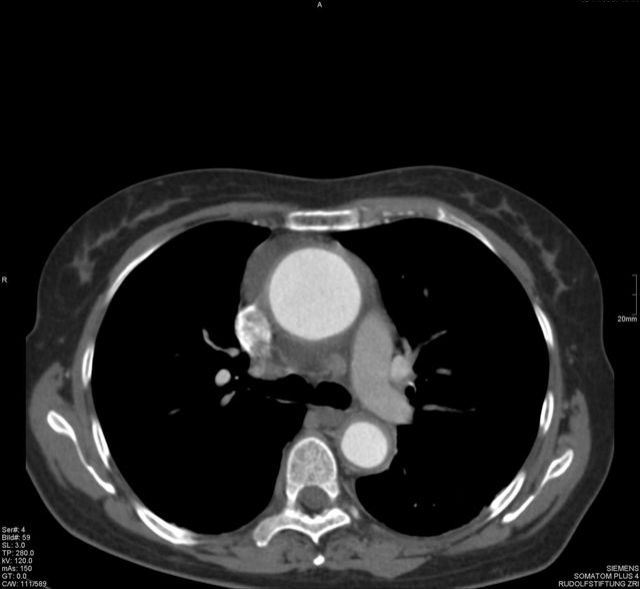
Thoracic CT from August 2004 - Thoracic CT from August 2004 showing an increase in the thickness of the aortic wall.
